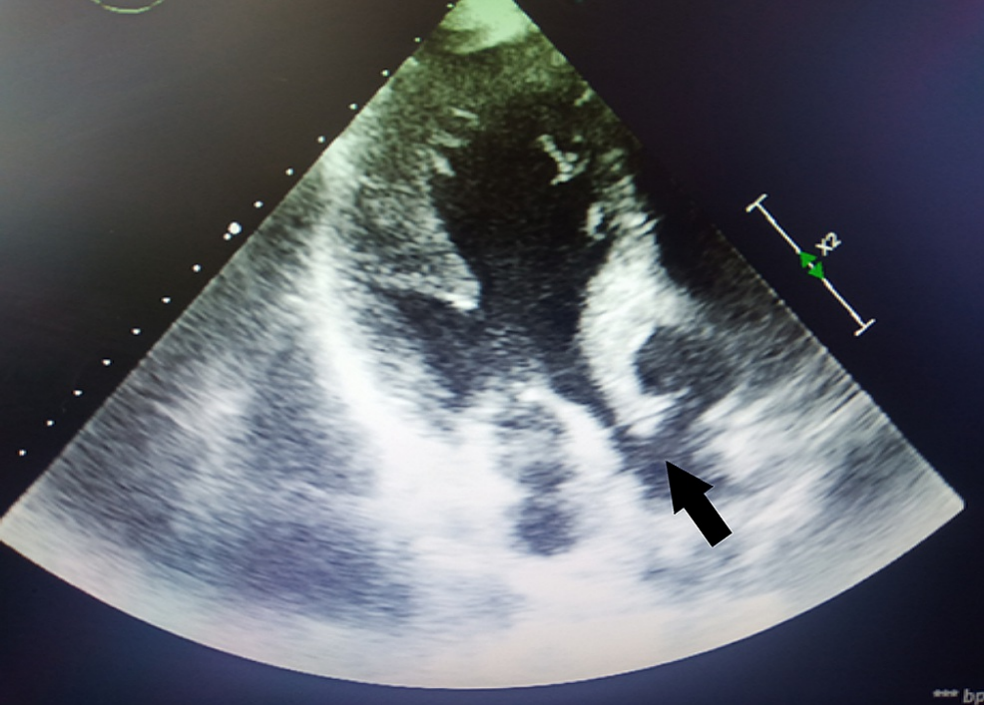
Transthoracic echocardiography. The parasternal long view shows the aneurysm sac with a compression effect to the left ventricular outflow tract.
